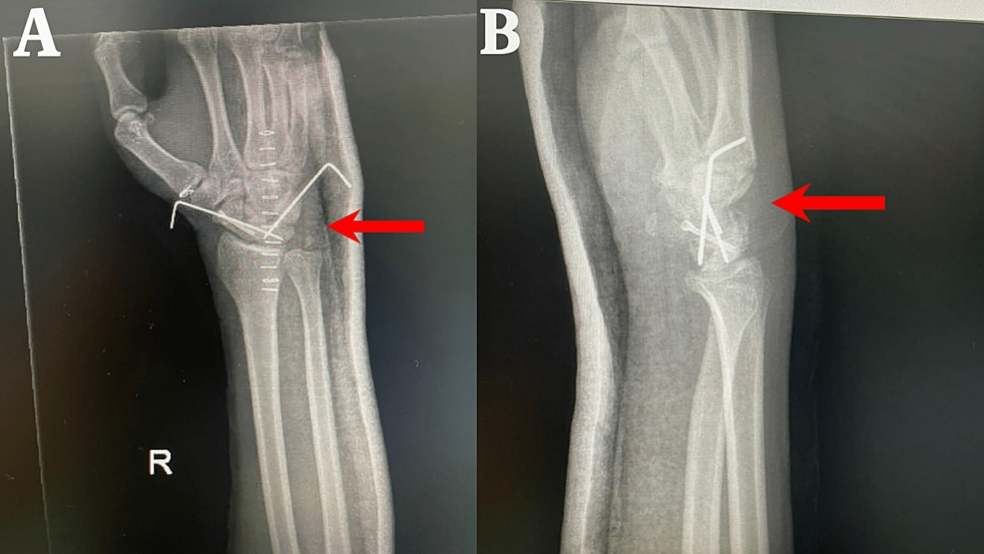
Postero-anterior (A) and lateral (B) views of post-operative x-ray showing Herbert screw and K wires in place
